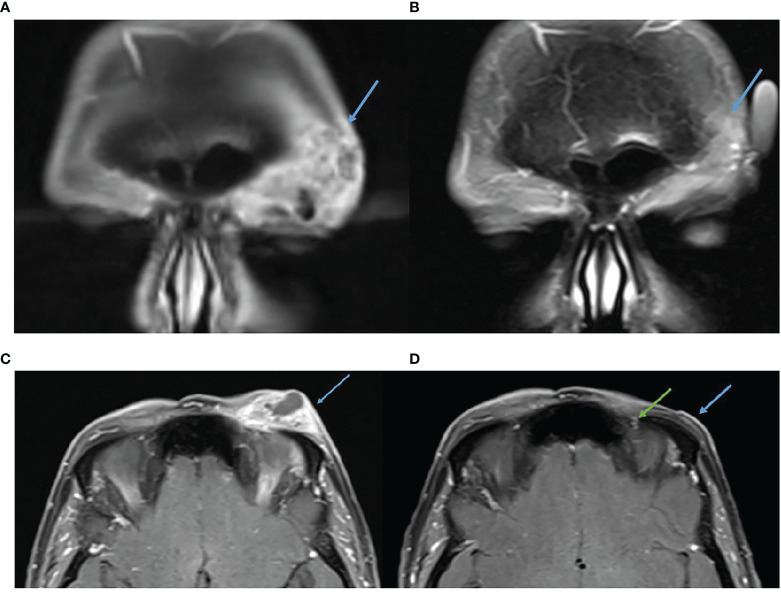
(A) Baseline, left, MRI post contrast T1 Fat Sat demonstrating 39 × 16 mm left forehead enhancing mass. (B) MRI post contrast T1 Fat Sat, post 16 cycles of cemiplimab 350 mg/3-weekly, demonstrating response. (C) Baseline, left, MRI post contrast T1 Fat Sat demonstrating 39 × 16 mm left forehead enhancing mass. (D) MRI post contrast T1 Fat Sat, post 16 cycles of cemiplimab 350 mg/3-weekly, no mass like enhancement and only small volume residual enhancement of the left supraorbital nerve, green arrow.
